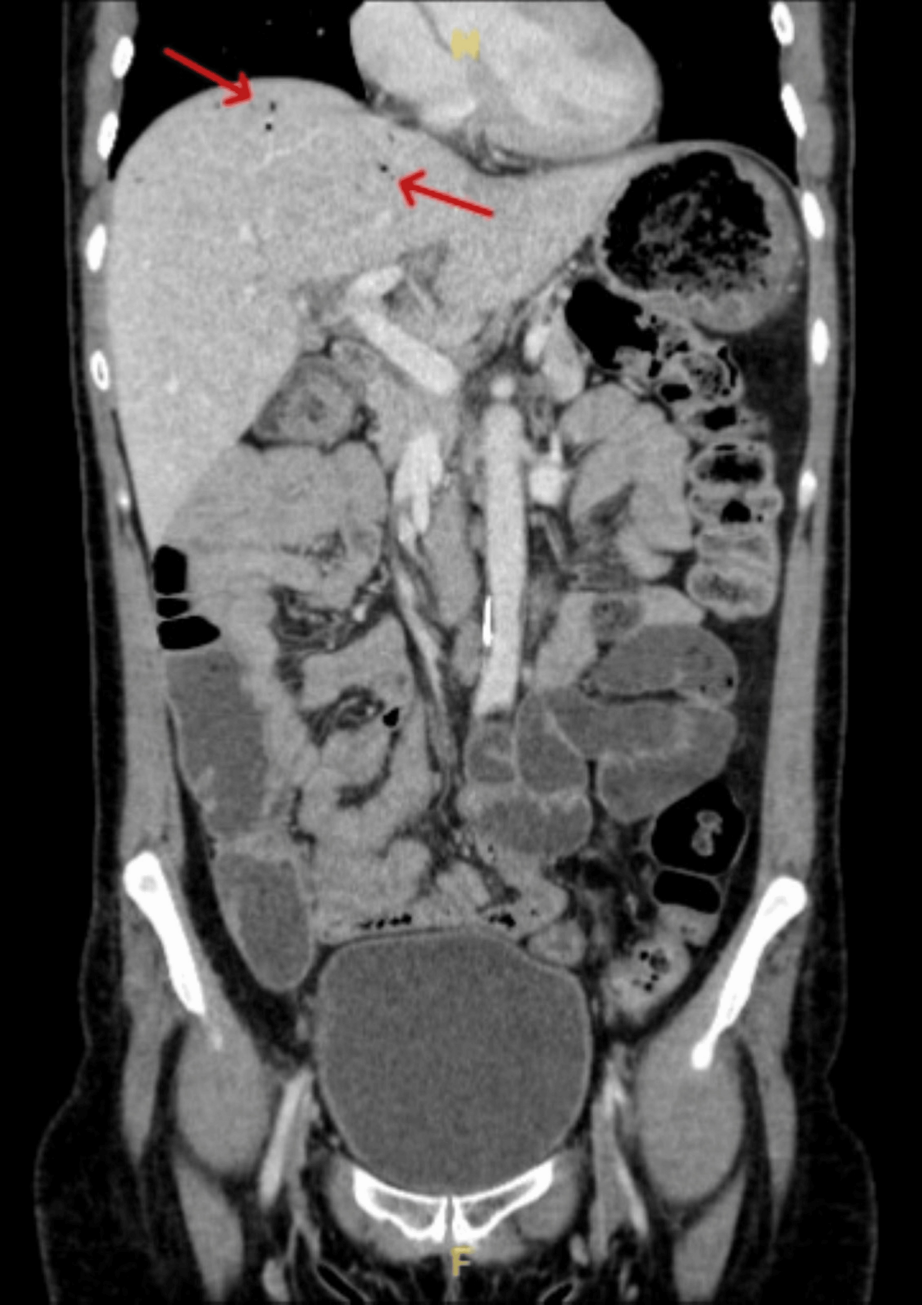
Coronal CT image demonstrating marked portal venous gas distributed throughout the periphery of the liver No pneumatosis intestinalis, bowel wall thickening, or intra-abdominal free air are seen. The remainder of the abdomen, including unprepared bowel loops, appears unremarkable CT: computed tomography
